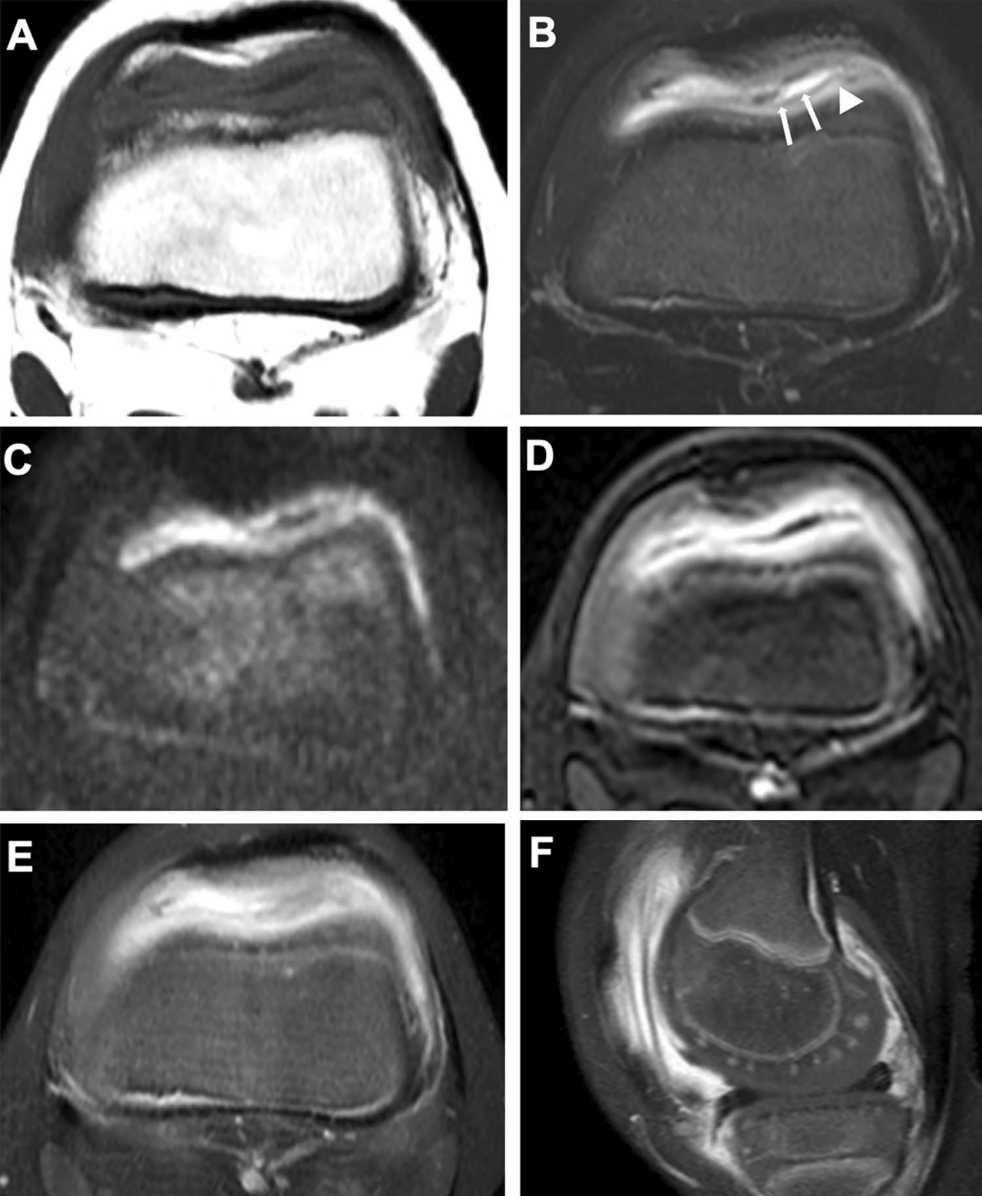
MRI in oligoarticular type JIA. A–F. A 4-year-old girl with painful left knee. T1-weighted image (A) and fat-suppressed T2-weighted images (B) of the left knee demonstrated thick synovial tissues. T2-weighted image reveals low signal of the superficial layer (arrows) and intermediate signal of the deep layer (arrowhead) of the thick synovium, contrasting with high signals of joint fluid (B). Axial DWI showed high-intensity synovium and low intensity joint fluid (C). Dynamic contrast enhanced axial image at the early phase showed prominent synovial enhancement (D). Contrast-enhanced fat-suppressed axial (E) and sagittal (F) T1-weighted image showed diffusion of contrast materials into the joint cavity and hyperemia (prominent vascular structures) in the epiphyseal cartilage
